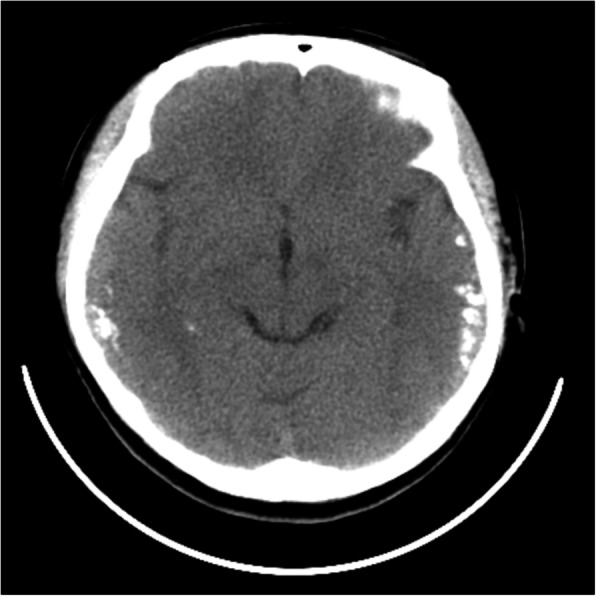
Plain computed tomography of the brain at third admission showing calcifications in bilateral temporal corticosubcortical areas were increased
